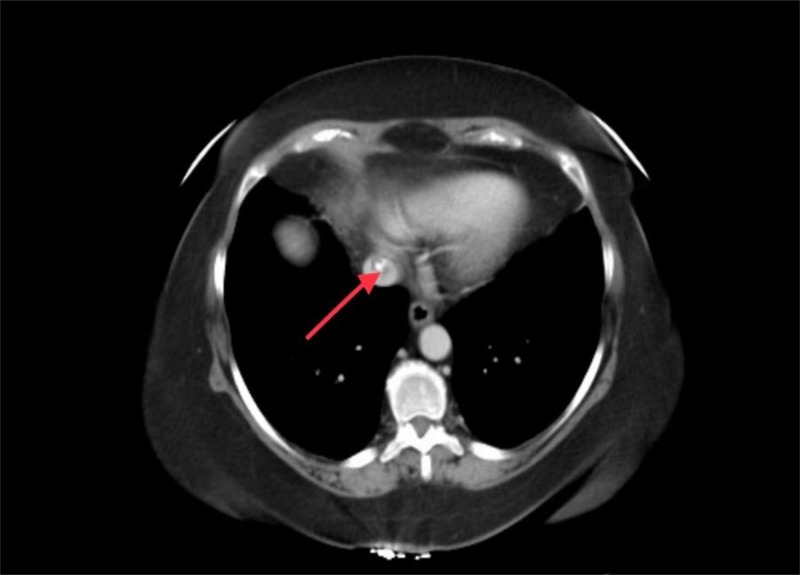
CT scan of the abdomen Calcified thrombus of the inferior vena cava (red arrow)
